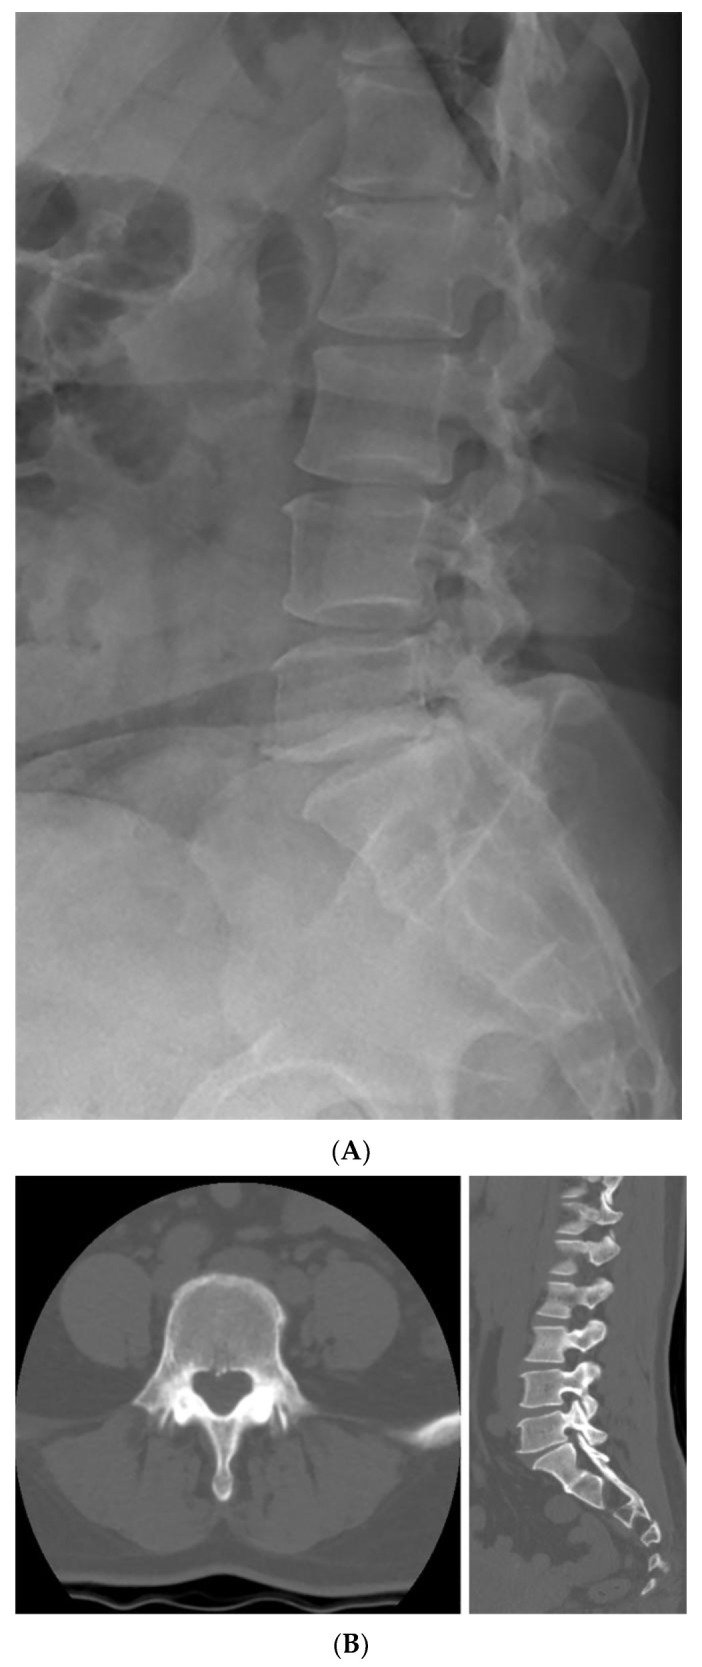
Four-month follow-up plain radiograph and CT scan after the injury. (A) The plain radiograph suggests almost complete bony union of the bilateral L5 pedicle fractures, although clear assessment of the fracture lines is limited. (B) The CT scan shows sclerotic change around the fracture margins and an interval reduction in the fracture lines.
